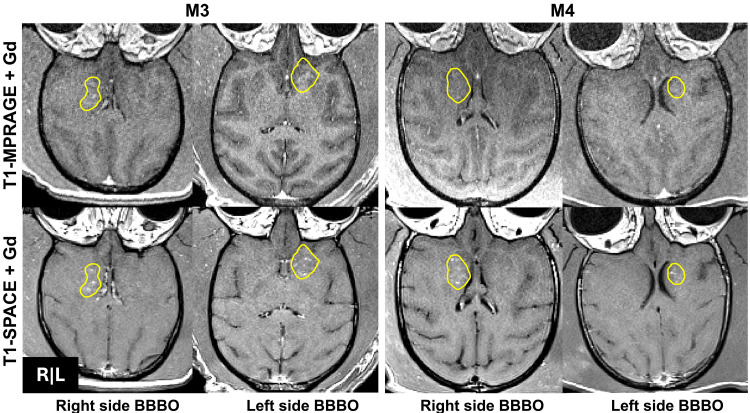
Enhanced BBBO Detection with T1-SPACE sequence. Representative postgadolinium T1-weighted MR images from M3 and M4 comparing the T1-MPRAGE (top row) and T1-SPACE (bottom row) sequences for detecting BBBO following LIFU + MBs targeting the right/left caudate. Yellow circles indicate regions of gadolinium enhancement, which are clearly visible in T1-SPACE but subtle and difficult to distinguish in T1-MPRAGE.
